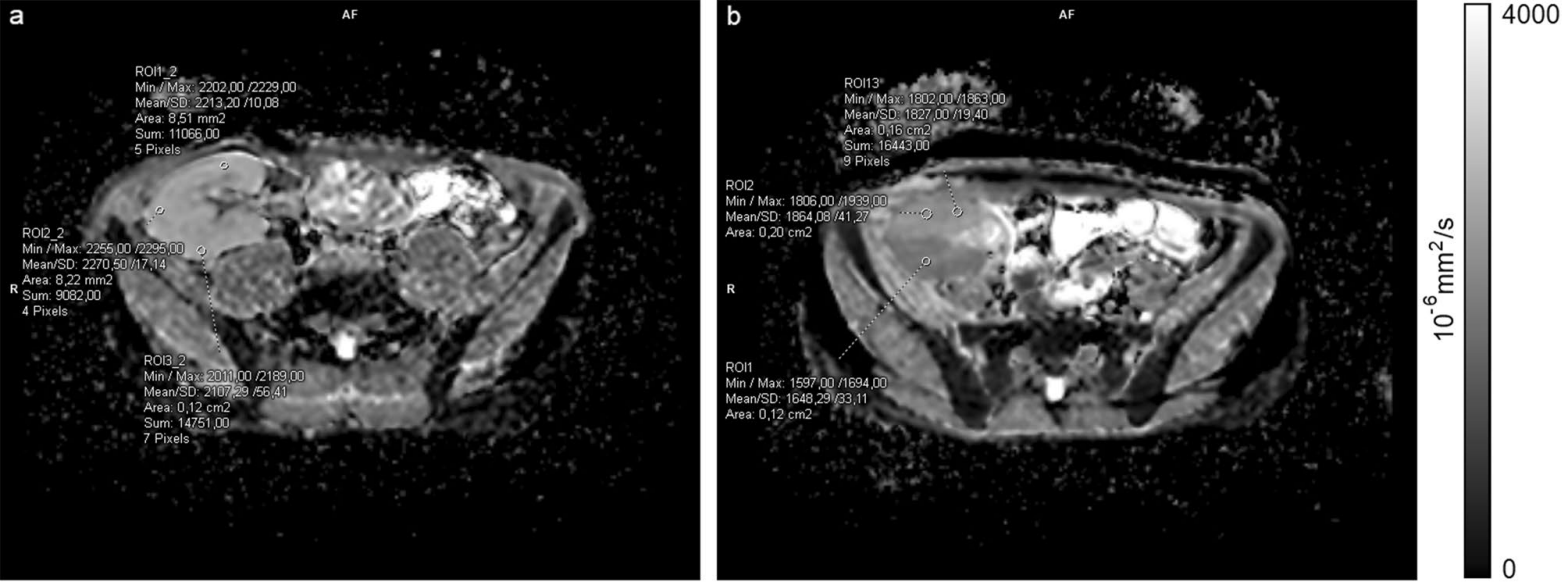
Axial ADC maps with representative circular regions of interest (ROI) placed in the renal cortex in: (a) patient with GFR ≥ 60 mL/min/1.73 m^2^ and (b) patient with GFR ≥ 60 mL/min/1.73 m^2^ (notice the difference in ADC values of anterior and posterior aspect of the kidney, with moderate diffusion restriction in posterior aspect after intraoperative vascular incident; this DWI appearance resolved after few months from transplantation).
